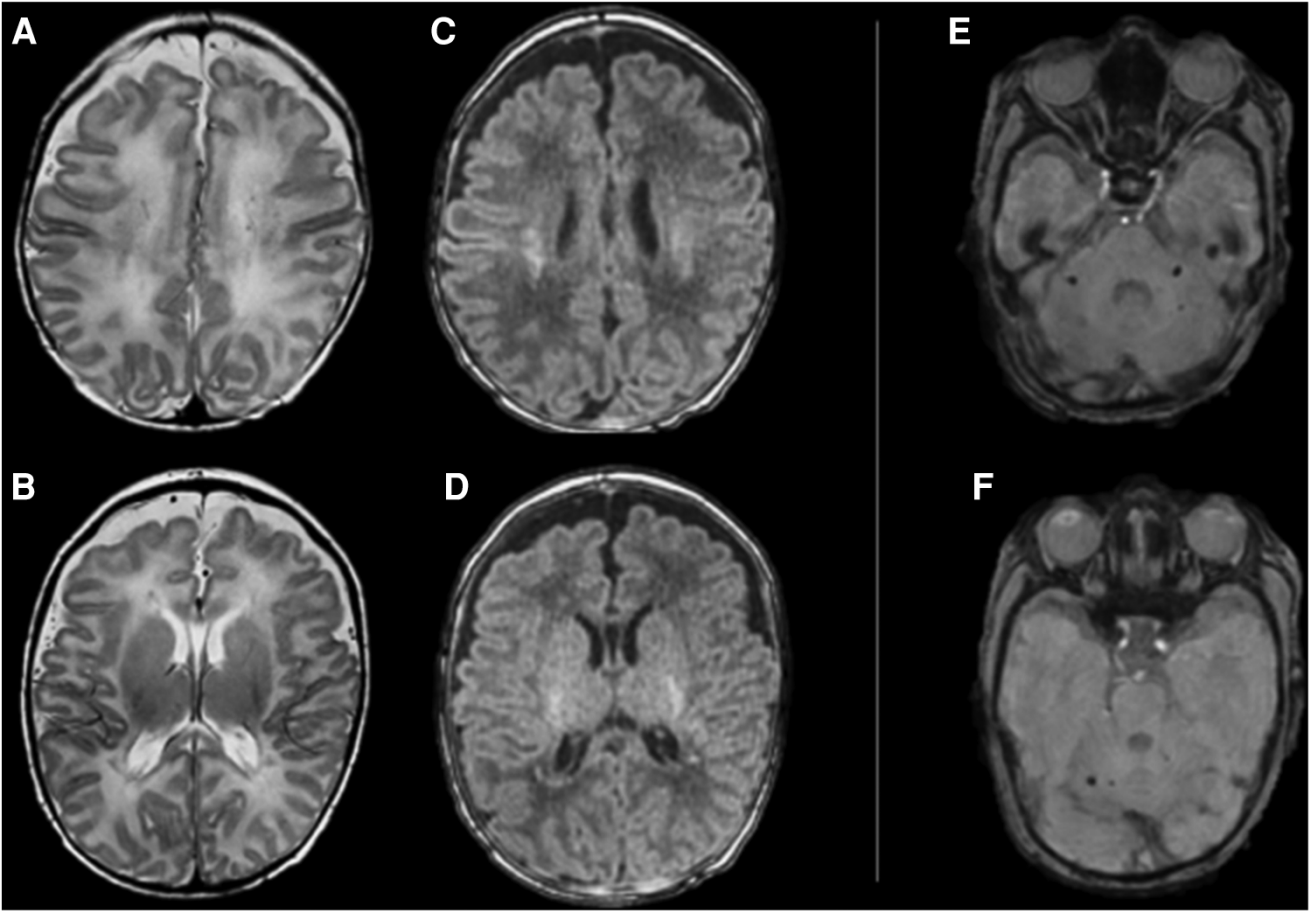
Brain MRI performed at 42 weeks of gestational age shows supratentorial (A–D) and infratentorial (E,F) pattern of brain injury. From left to right, supratentorial axial FSE T2 images (A–D) showing hypointense punctate confluent white matter lesions in the centrum semiovale (A) and in the periventricular white matter (B); axial MP-RAGE T1 images showing hyperintense signal corresponding to the abovementioned T2 signal abnormalities (C,D). Infratentorial axial Susceptibility Weighted images (E,F) shows rounded small spots of signal intensity in the cerebellar peduncles bilaterally (E) and in the right dentate region (F).
